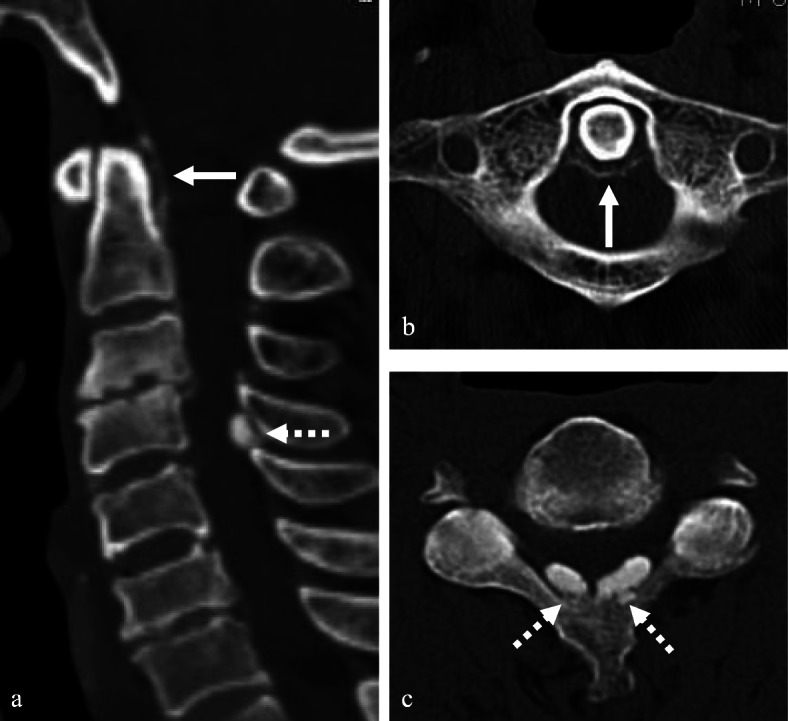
Sagittal and axial CT scans revealed calcified lesion behind the dens (a and b) (white arrows) and spinal cord compression by calcified round lesions (a and c) at C4 (dotted white arrows)
